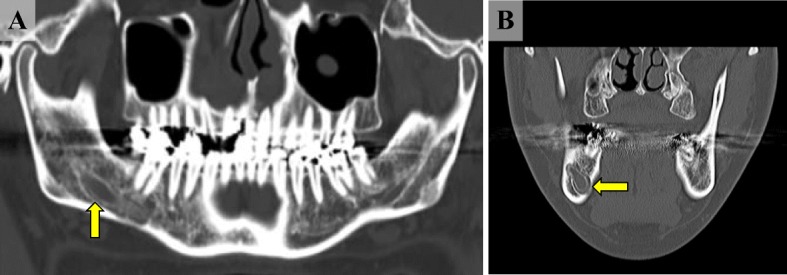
X-ray findings. a X-ray CT finding (panoramic image). A radiolucent finding of a similar circle was recognized in the right mandible body (yellow arrow). b X-ray CT finding (coronal image). We confirmed the enlargement of the right mandible canal. The destruction of the mandibular canal wall is not clear (yellow arrow)
